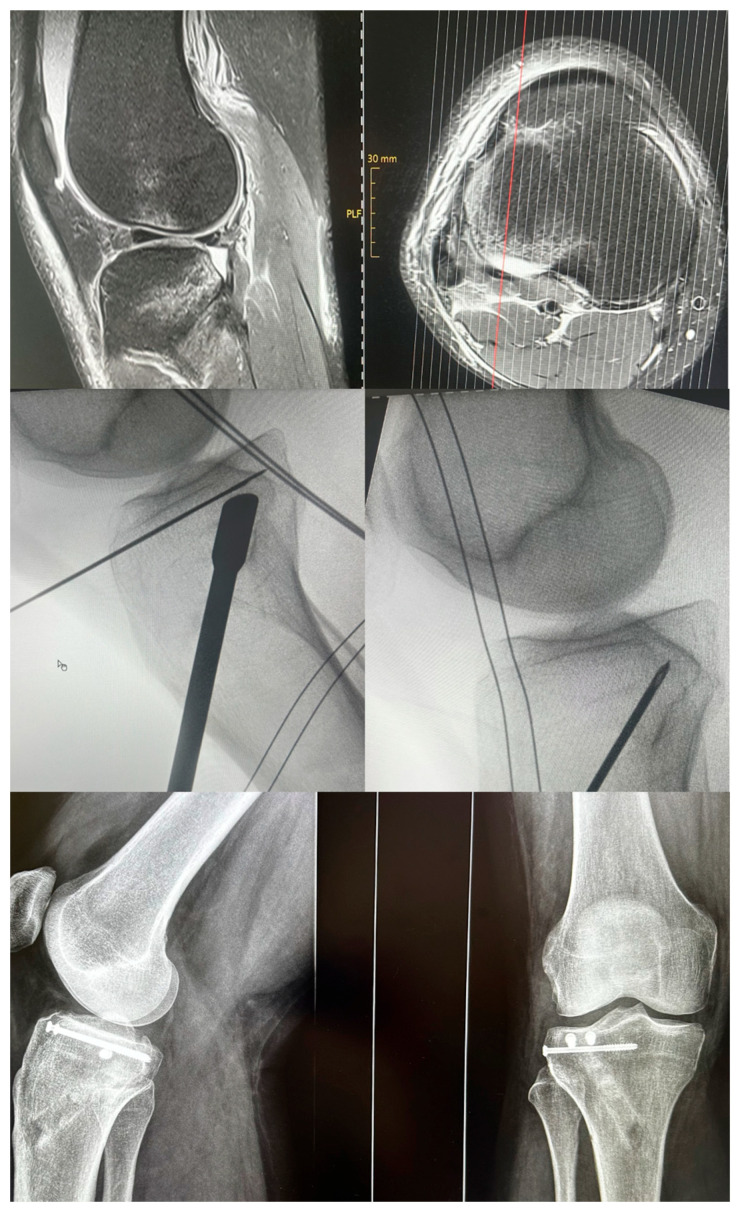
Preoperative MRI images and intraoperative radiographs of a female patient with an anterior cruciate ligament rupture and a posterolateral TPF. Shown is an example of surgical management using a minimally invasive, indirect reduction technique, screw osteosynthesis in a jail technique, and defect filling with allogeneic cancellous bone graft.
